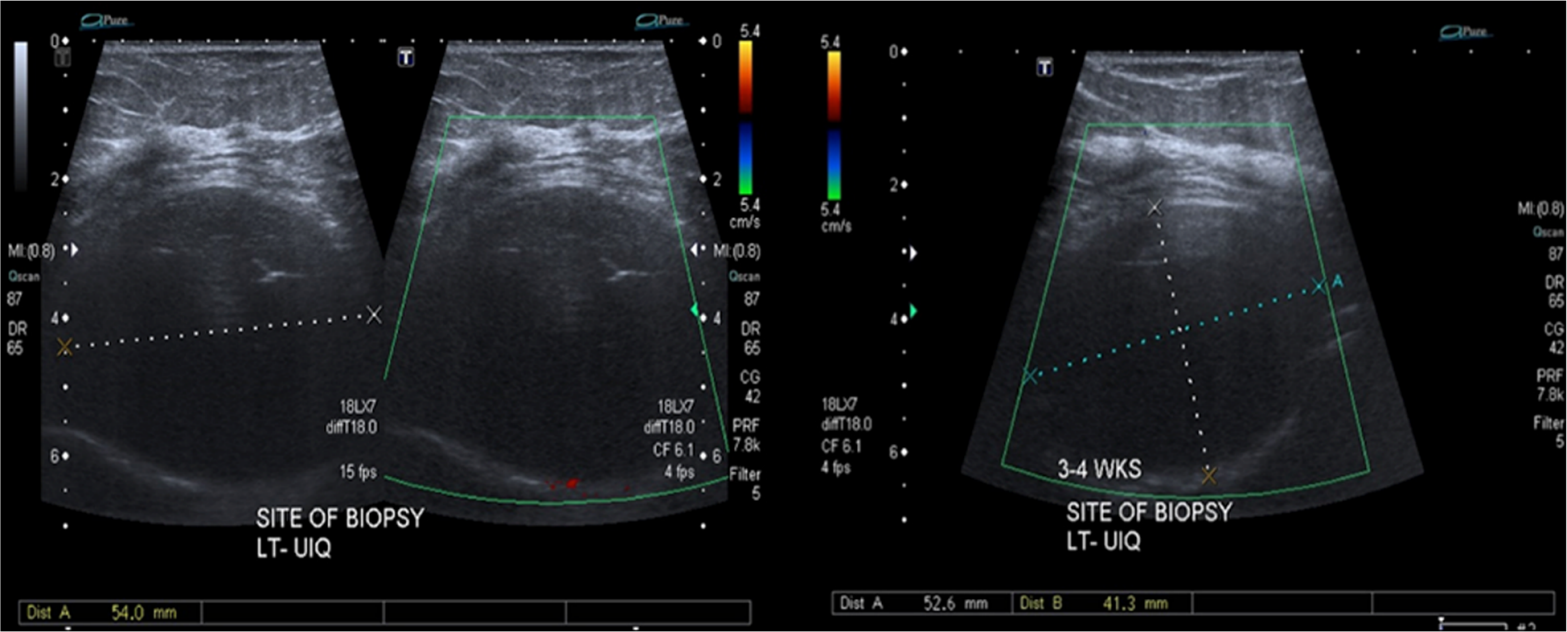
Grayscale and Doppler ultrasound of the left breast exhibit a large (5.2 × 4.2 × 5.4 cm) hypoechoic/anechoic lesion located at the left retro-pectoralis region with no evidence of vascularity
